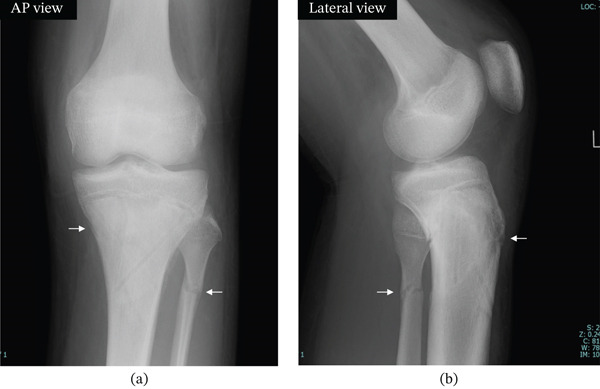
Plain x‐ray images of the left knee at 9 weeks postoperatively. (a, b) Radiographs of the left knee joint at 9 weeks postoperatively. The fracture lines are indicated by arrows. Both the left tibia and left fibula show good bone union, with no signs of displacement: (a) AP view and (b) lateral view.
